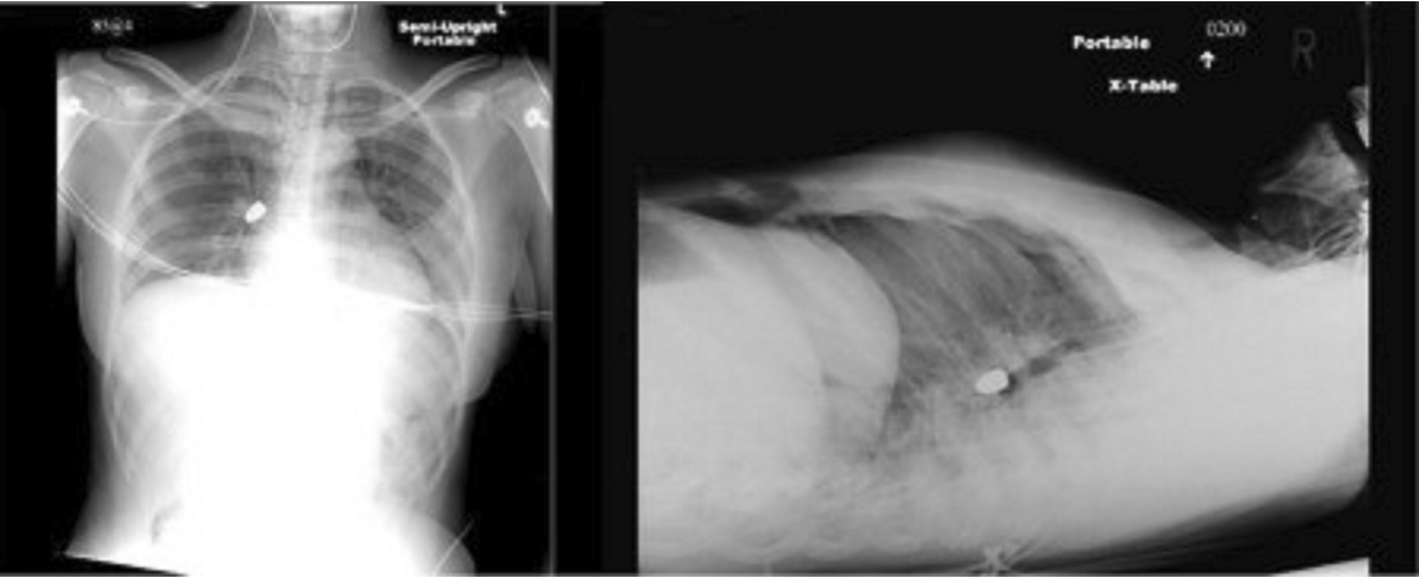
Chest X-ray showing a bullet located within right paracardiac position.
